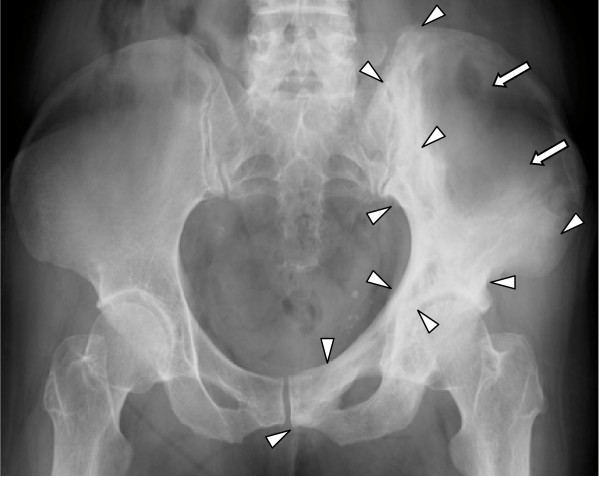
Radiography of patient 2 showed pagetic changes as osteolytic (white arrow) and sclerotic (white arrowhead) changes in the left acetabulum, half of the ilium, and the pubic bone.
